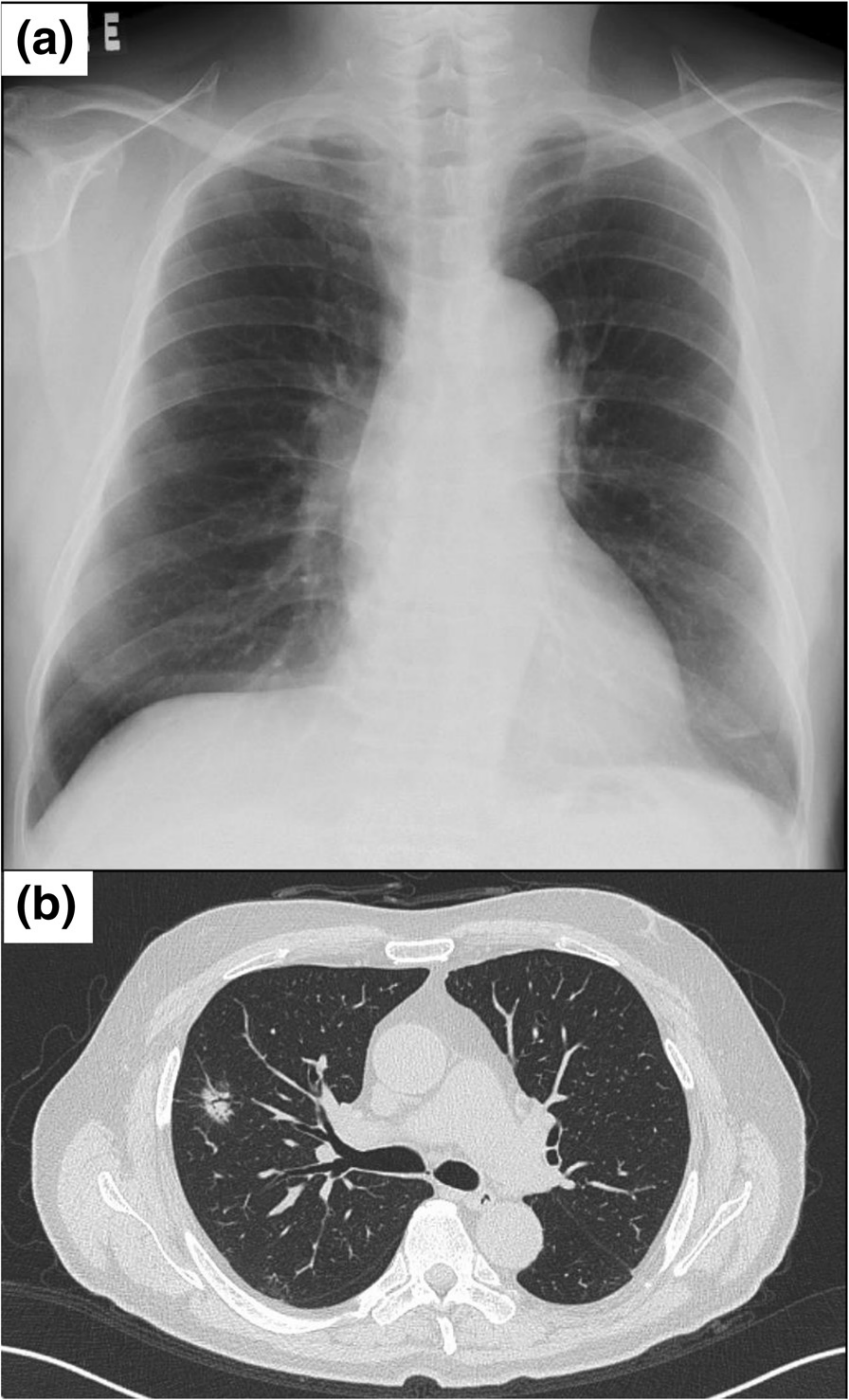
The findings of a chest radiograph and computed tomography. a Chest radiograph shows a nodular shadow in the middle lobe of his right lung. b Chest computed tomography revealed a 20-mm spiculated part-solid nodule with pleural indentation in segment 3 on the right
